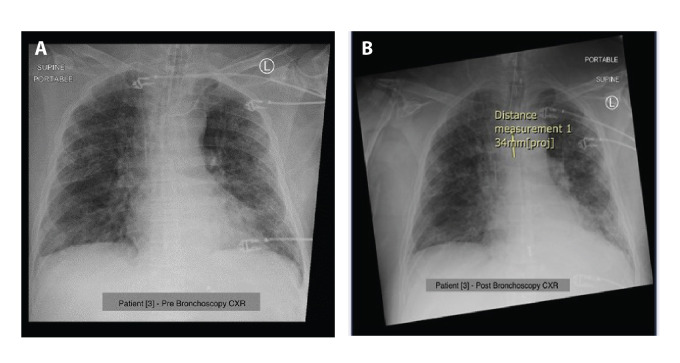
Chest radiograph for patient 3 (A) pre-and (B) post-bronchoscopy
